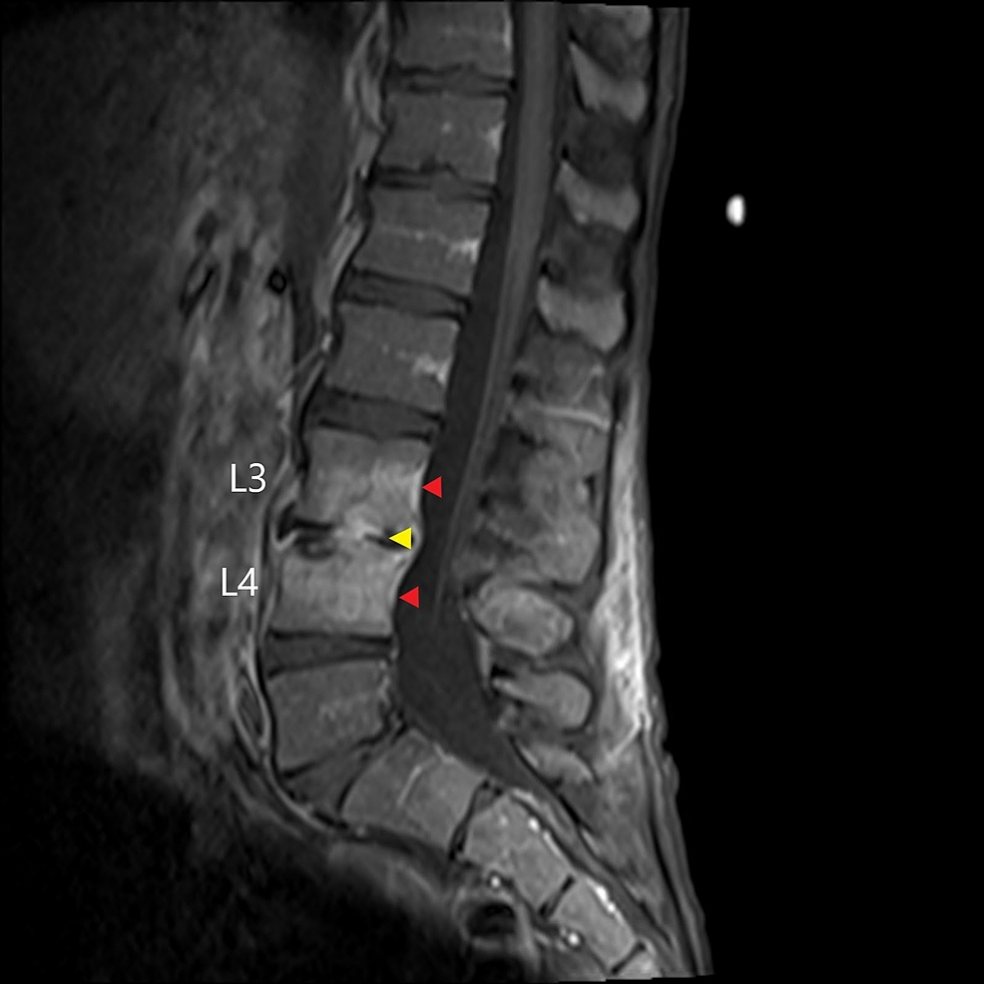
Post-treatment T1 fat-suppressed post-gadolinium MRI sagittal view demonstrating enhancement of the L3/L4 disc space (yellow arrowhead) and enhancement of the adjacent L3 and L4 vertebral bodies (red arrowheads). MRI: magnetic resonance imaging
